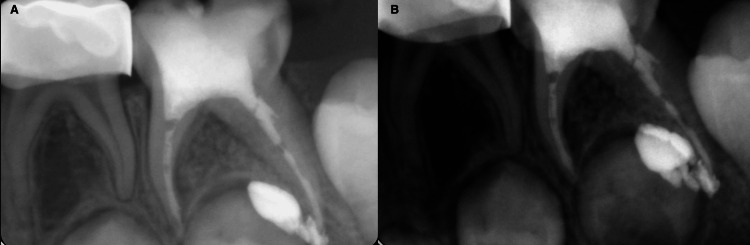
Immediate postoperative PA radiograph shows extruded Vitapex related to the D canal A: Immediate periapical radiograph after pulpectomy; B: Another immediate periapical radiograph after pulpectomy from different angle
